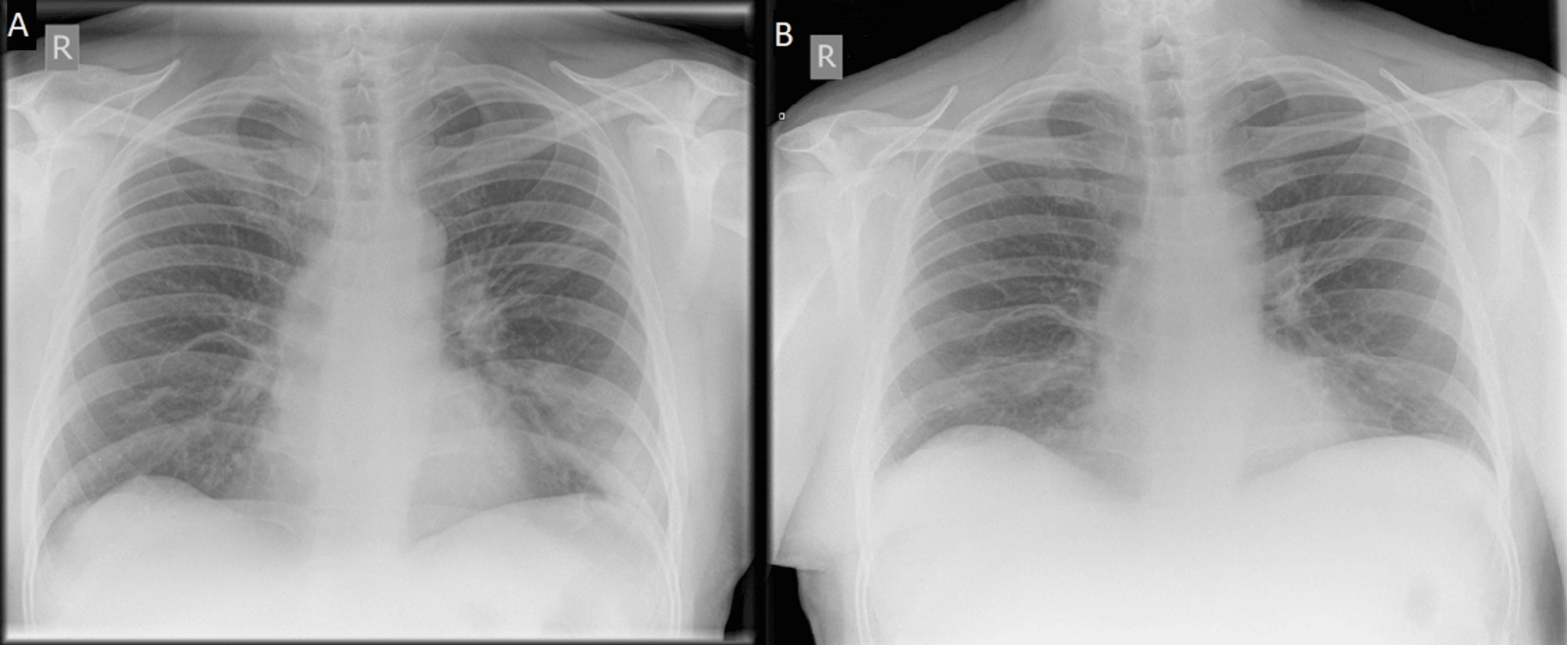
(A) PA chest x-ray of our patient showing shadowing in the left lower lobe. (B) Repeat PA chest x-ray showing the resolution of the shadowing in the left lower lobe following treatment. Signed consent was obtained from the patient prior to using the images in the case report.
